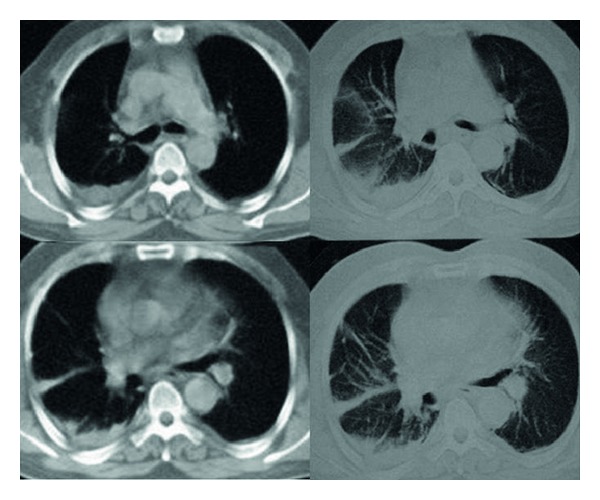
Thorax computed tomography showing the bilateral pleural effusion and linear atelectasis.
